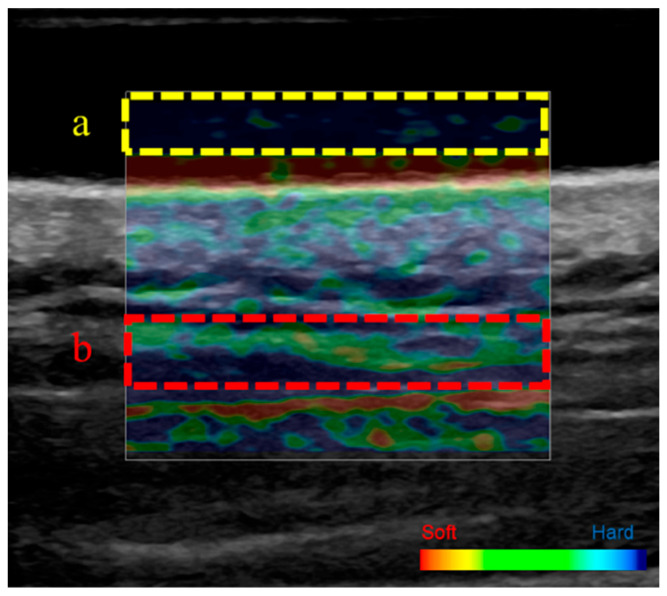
Ultrasound strain elastography image and regions of interest (ROIs). ROI of the acoustic coupler gel (a) and ROI of the upper trapezius muscle (b). Red indicates softer areas, whereas blue indicates harder areas.
